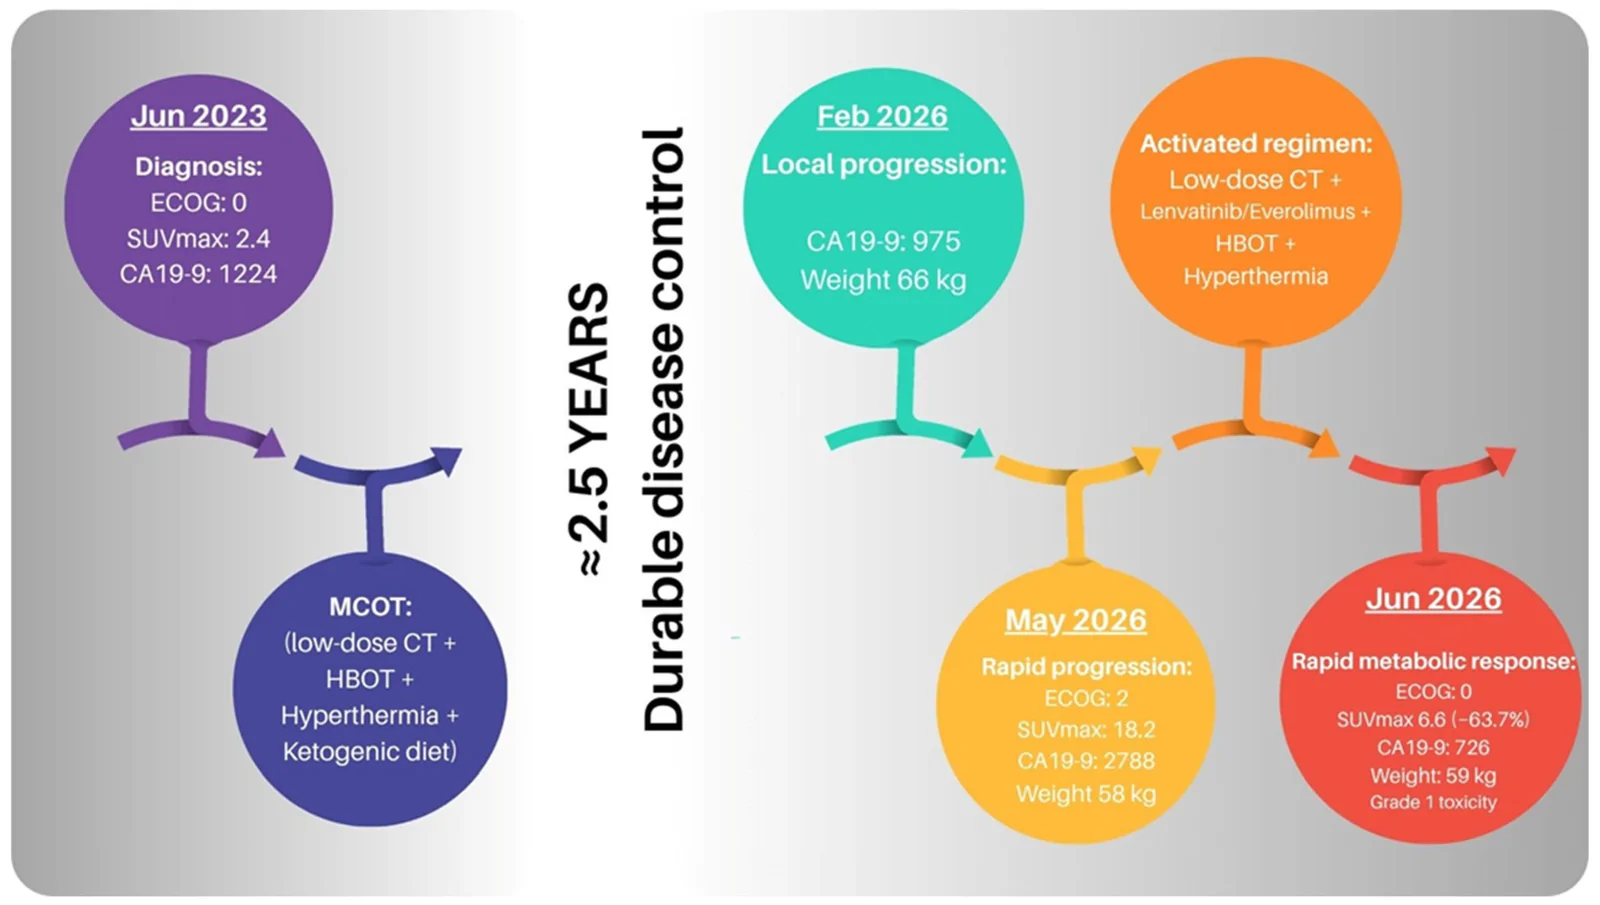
Clinical timeline and metabolic response following activated multimodal therapy. LA, locally advanced; PDAC, pancreatic ductal adenocarcinoma; MCOT, metabolically controlled oncologic therapy; CT, chemotherapy; HBOT, hyperbaric oxygen therapy.
